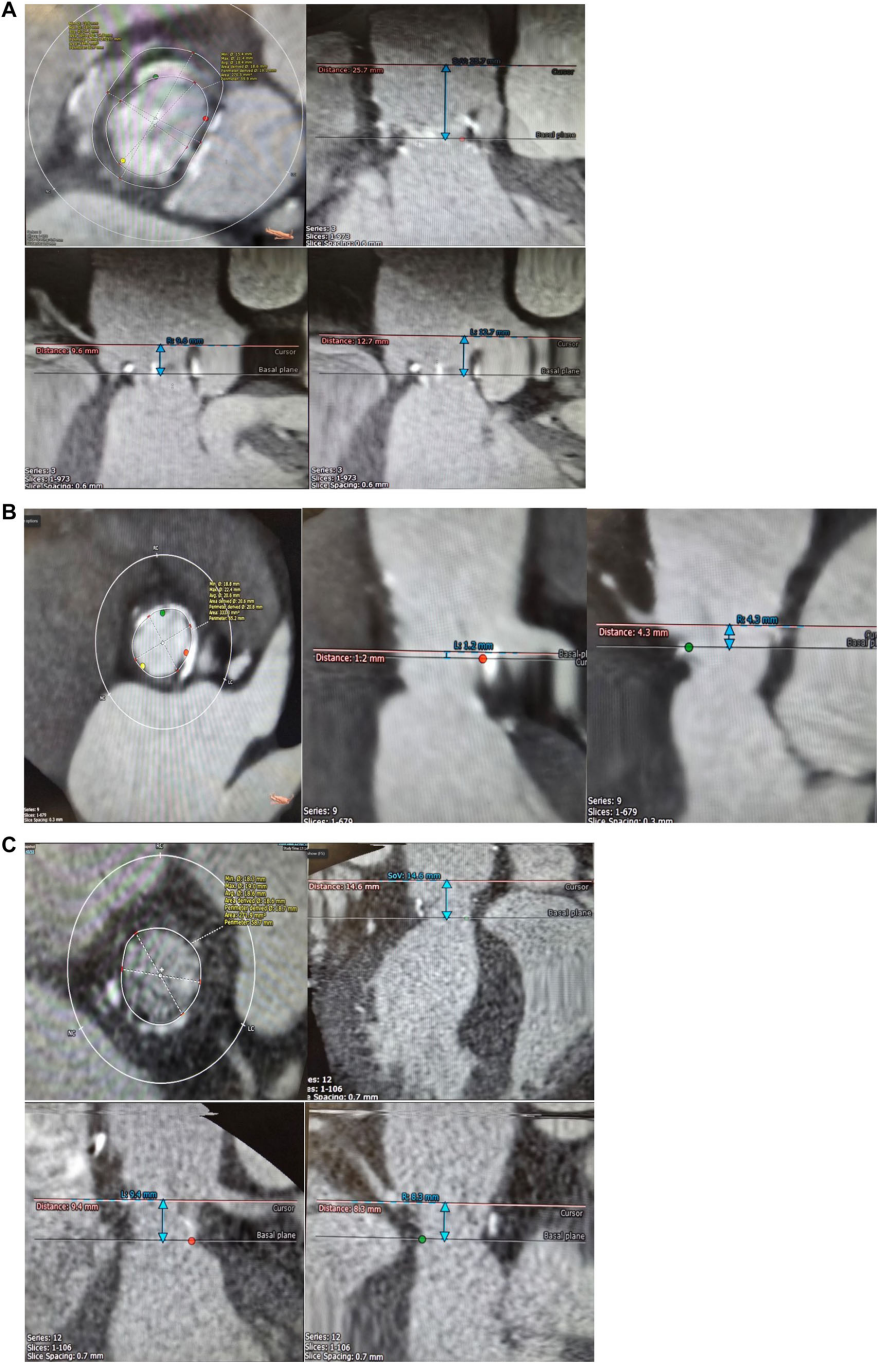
(A) Showing CT scan 3mensio reconstruction of annulus for patient 1. (B) Showing CT scan 3mensio reconstruction of annulus for patient 2. (C) Showing CT scan 3mensio reconstruction of annulus for patient 3. [Color figure can be viewed at wileyonlinelibrary.com]
